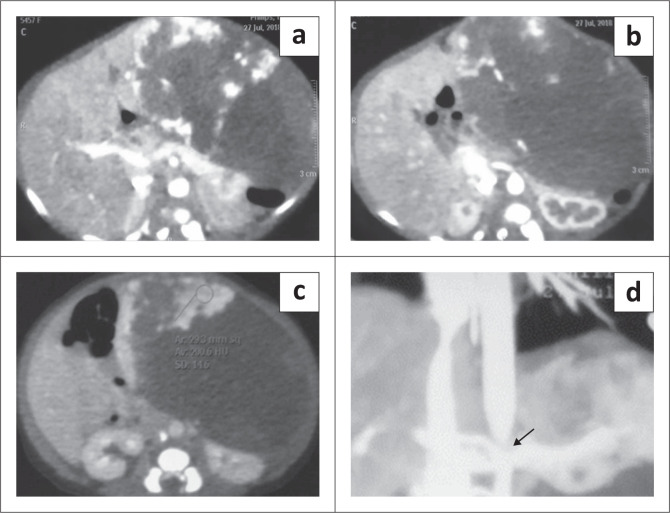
A 10-day-old female with a congenital hepatic haemangioma presented with left-sided abdominal swelling. Multiphase contrast-enhanced CT abdomen revealed a large, necrotic, exophytic mass lesion in the left lobe of the liver, almost completely replacing it. The lesion shows peripheral nodular enhancement in the arterial phase (a and b) with a large non-enhancing area. There is centripetal enhancement; however, the central portion remains hypodense even on the delayed phase (c). Note the attenuation of the abdominal aorta distal to the origin of the coeliac axis on the coronal maximum intensity projection image (arrow in d).
